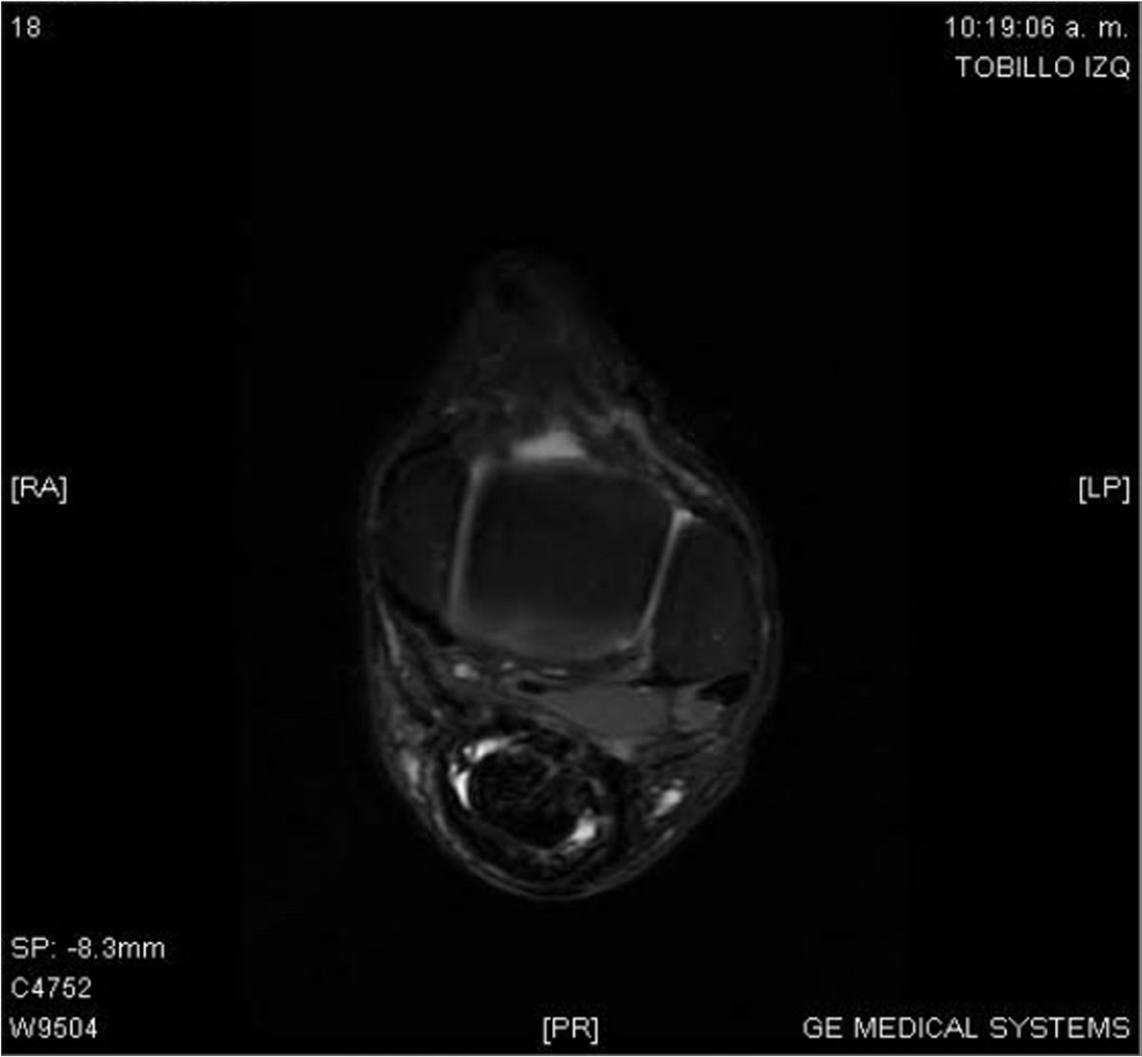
Transverse plane MRI of left ankle - hypointense Achilles tendon with fusiform appearance, enhanced by Karger's fat, hyperintense on T1 and T2 with fat saturation
